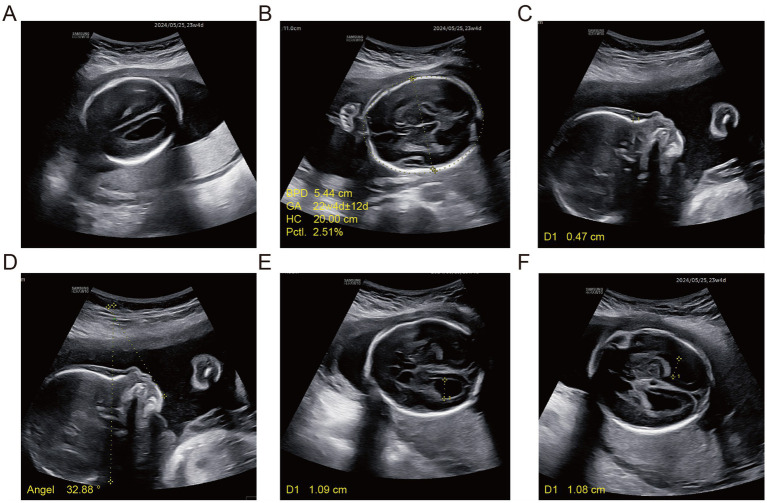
Fetal ultrasonography findings. (A) Bilateral parieto-occipital sulci are not visualized on the transcranial axial view. (B) Transthalamic axial plane: BPD (5.44 cm) and HC (20.00 cm) both < expected for gestational age. (C) Thickening soft tissue. (D) Frontal soft tissue thickening with a mandibular-facial angle <49° and micrognathia. (E) Right lateral ventricle posterior horn 10.9 mm (far-field standard plane). (F) Left lateral ventricle 10.8 mm (non-standard near-field imaging plane).
